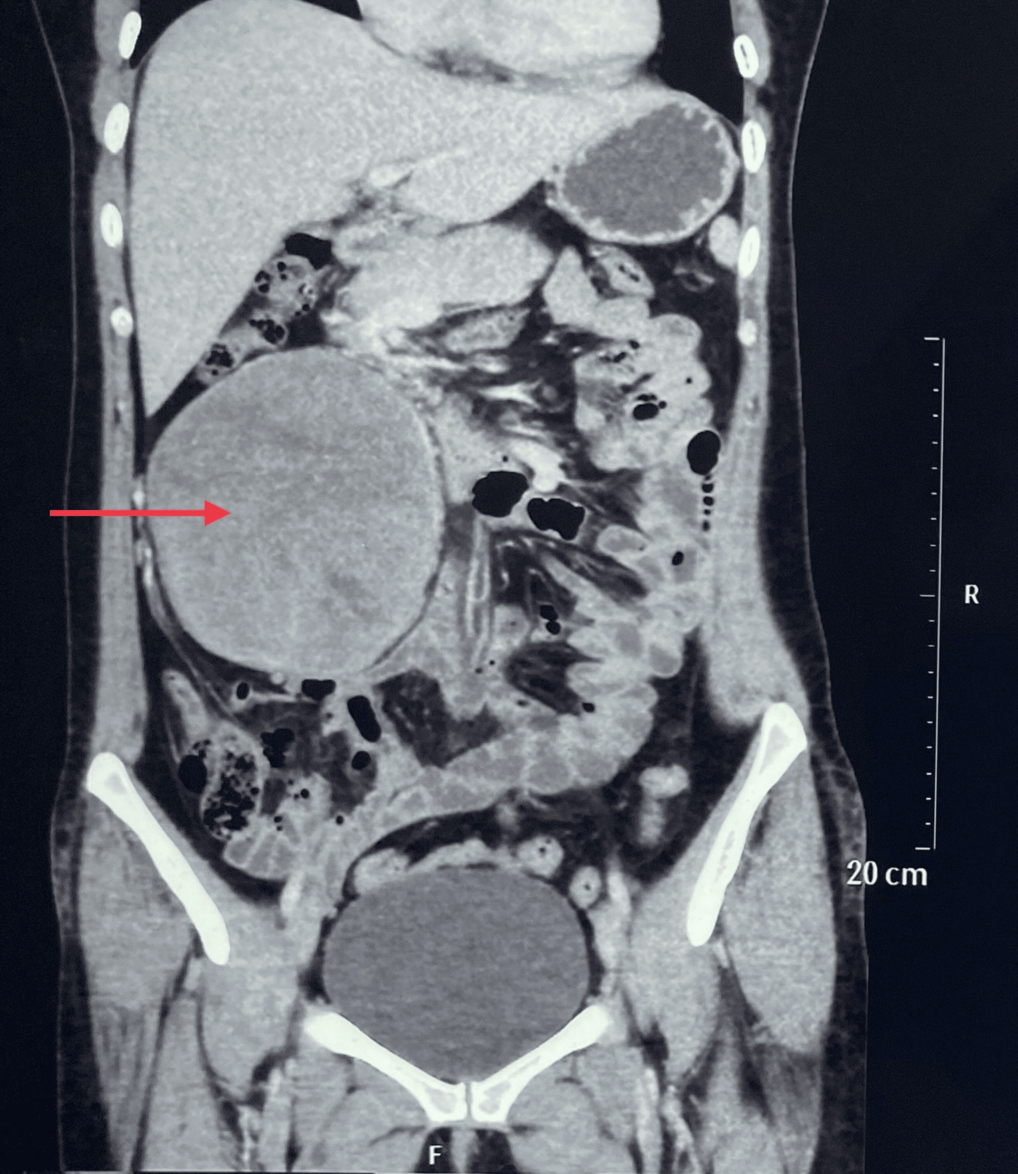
CECT abdomen coronal section showing a heterogeneously enhanced well-defined soft tissue density mass in the right hypochondriac and lumbar region (red arrow). CECT: Contrast-Enhanced Computed Tomography
